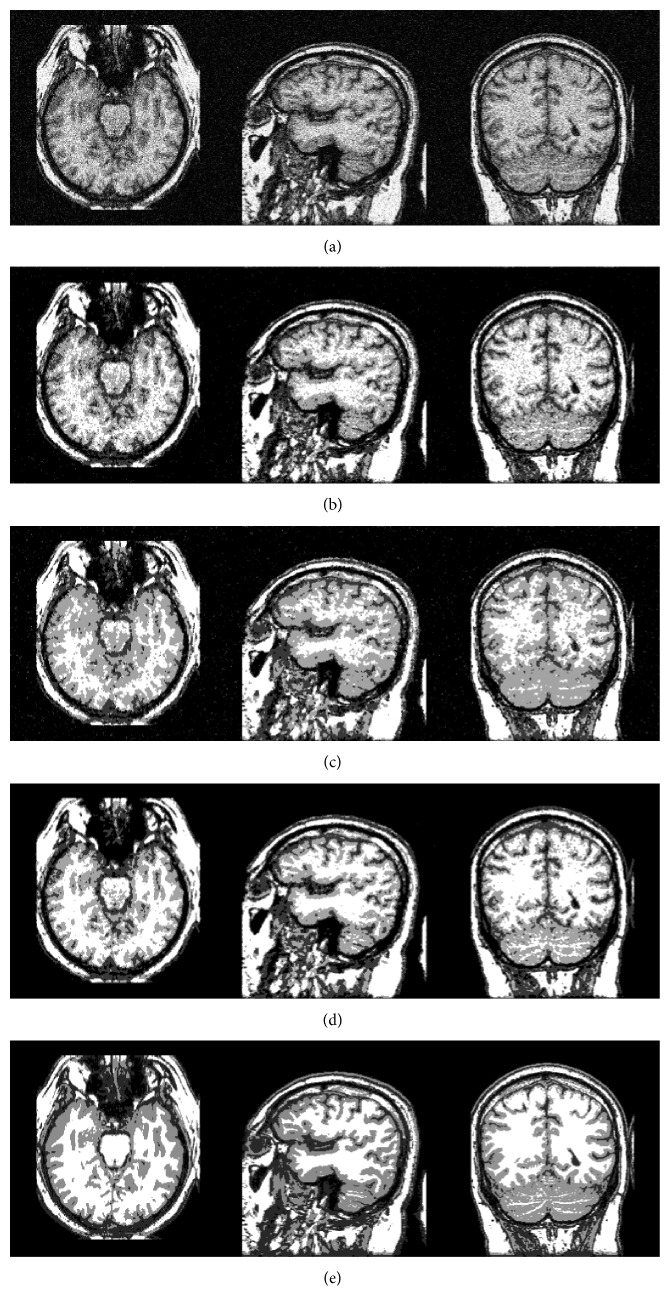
Experimental results on real MR image: (a) three original images, (b) BCFCM, (c) HMRF-EM, (d) SCFCM, and (e) our method.
